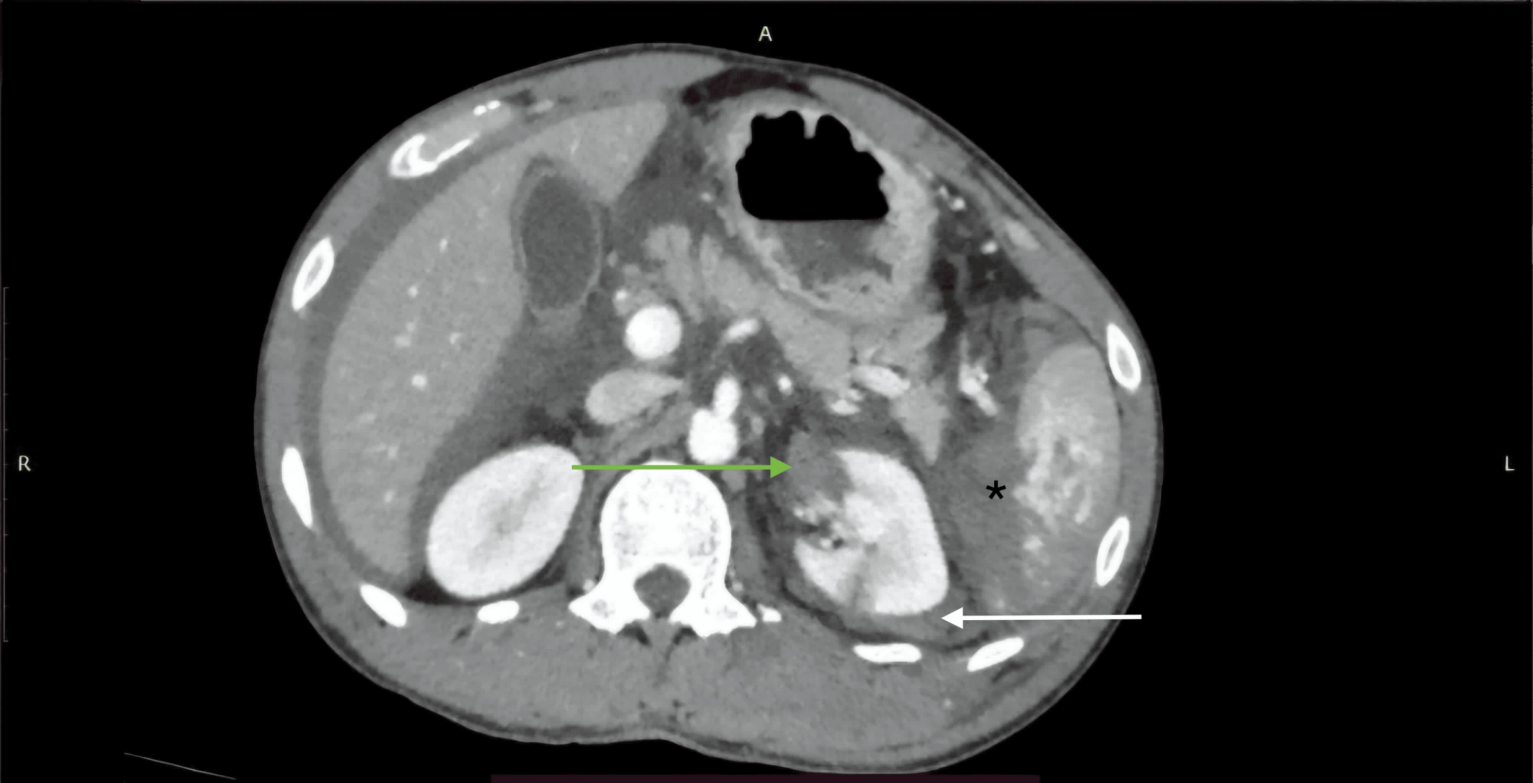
AAST grade III renal injury with wedge-shaped laceration and grade IV splenic injury with devascularization on CECT (SB protocol). A 32-year-old male with a history of RTA underwent CECT of the abdomen using the SB protocol. The axial CECT image reveals a 1.2 cm wedge-shaped laceration affecting the upper pole of the left kidney (green arrow), accompanied by a perirenal hematoma (white arrow). Additionally, a large splenic parenchymal laceration extending up to the hilum with approximately 40% devascularization of the splenic parenchyma (black star) and moderate hemoperitoneum is noted. Findings were consistent with the AAST grade III renal injury and grade IV splenic injury. AAST: American Association for the Surgery of Trauma; CECT: contrast-enhanced computed tomography; SB: split-bolus; RTA: road traffic accident.
